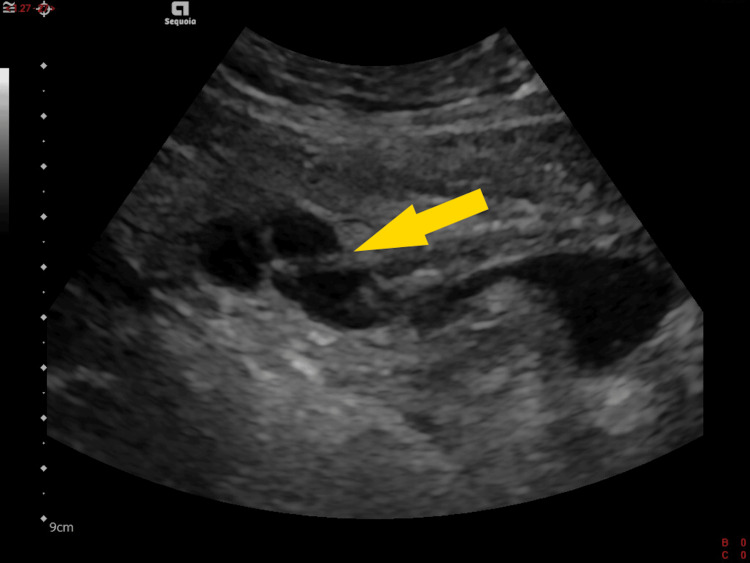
Sagittal section of US showing the urachal cyst (arrow) to be located in front of the bladder and divided into three portions as a result of the cross-sectional effect US: ultrasound
